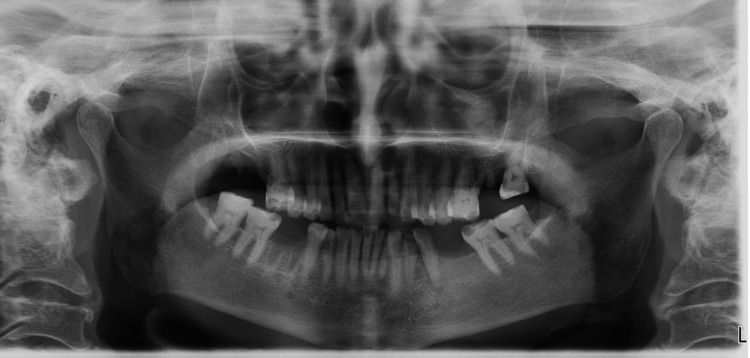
Orthopantomography revealed generalized attrition and missing right and left mandibular first molars, as well as the right maxillary second molar
